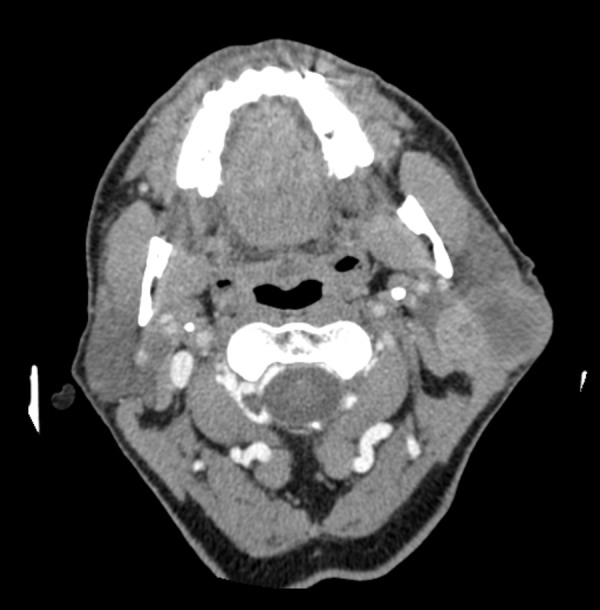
An enhanced CT scan of the parotid gland. Heterogeneously enhanced, left parotid mass, involving both lobes of the gland.
